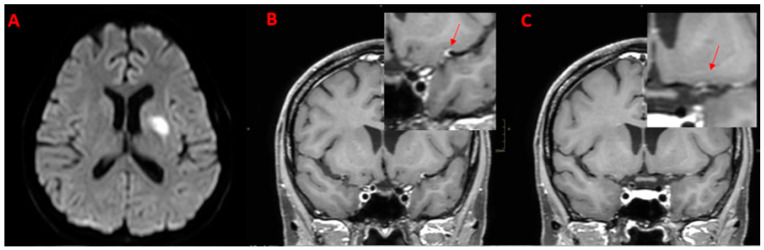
(A–C) Patient (F/39) with frequent episodes of speech impairment and arm and limb stiffness. The diagnosis was of CNS HIV-related vasculitis. (A) Axial DWI image shows a left nucleo-capsular hyperintense area with restricted diffusion. (B,C) Coronal BB T1w VWI after administration of the contrast agent shows an intense, concentric wall enhancement (arrow) of the left middle cerebral artery.
